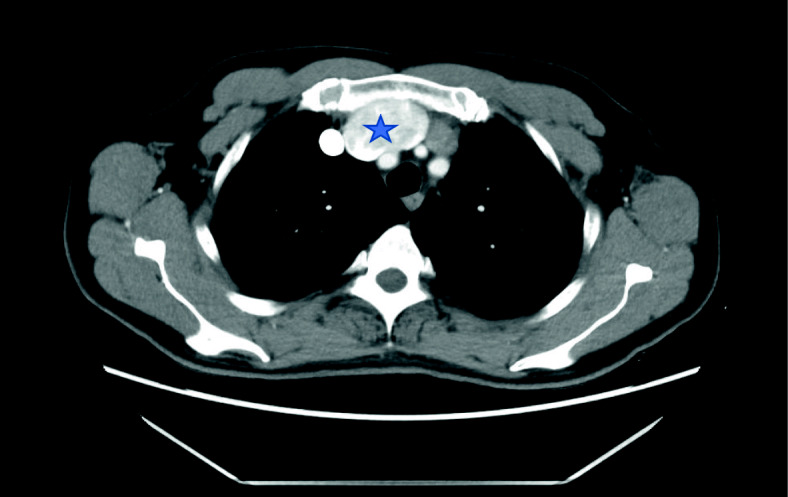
Chest-enhanced CT scan showed the location of the vein aneurysm, marked with a pentacle
